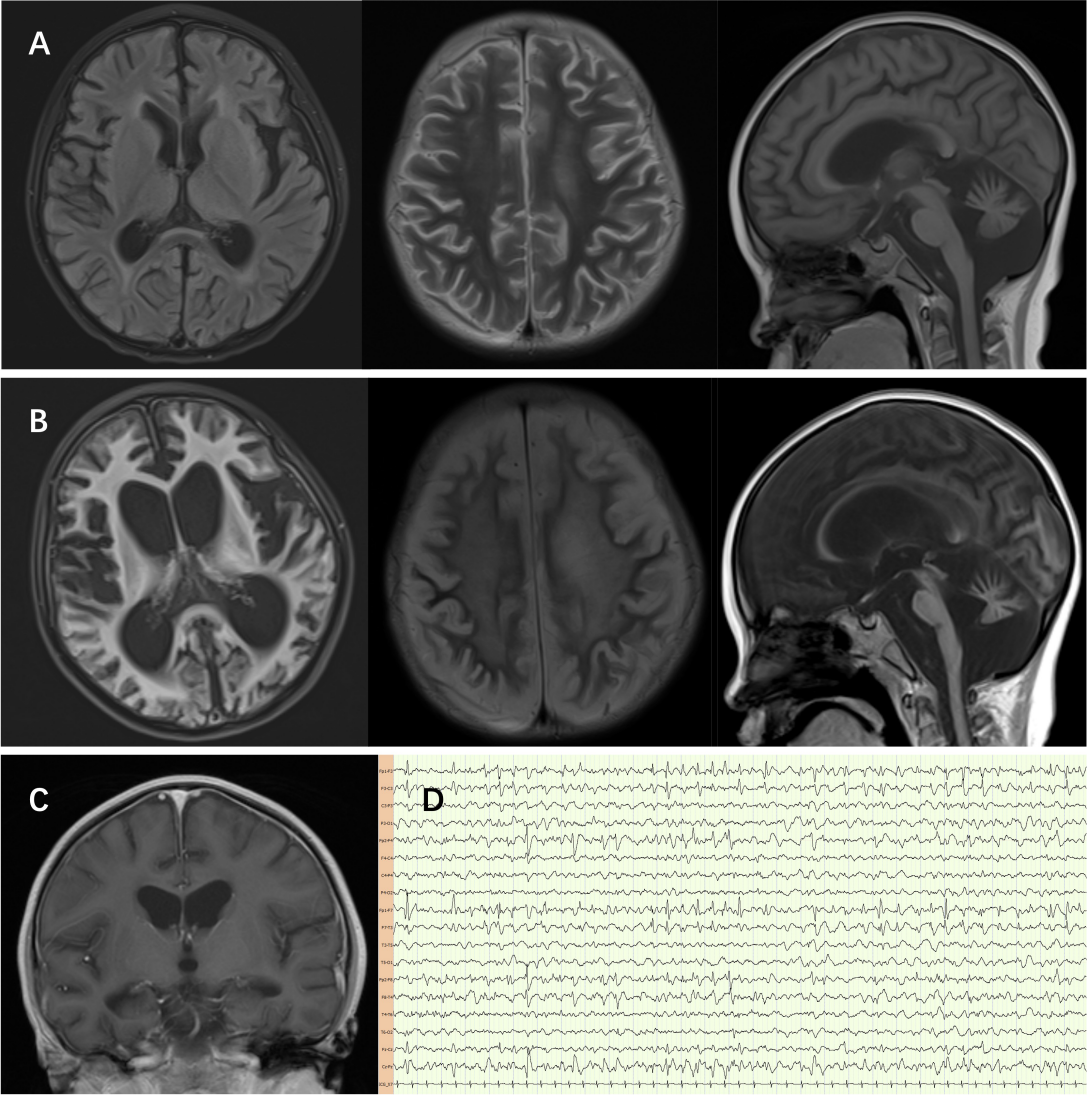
(A) The sulci of both cerebral hemispheres and cerebellar hemispheres are deepened, the gyri are thin, the white matter is reduced, the extracerebral space is widened, the ventricles are full, the cisterna magna is widened, and patchy FLAIR slightly high signals can be seen in the frontal, temporal, insular lobes and the anterior and posterior horns of the lateral ventricles. The FLAIR signal along the sulci and gyri of the cerebellum is slightly high, and the T2 and FLAIR signals of the right thalamus are slightly high. (B, C) Diffuse atrophy of the cerebrum and cerebellum worsened after 1 year follow-up. (D) Electroencephalography revealed interictal bilateral frontal and temporal spike-and-wave discharges, no periodic discharges nor epileptic seizures.
